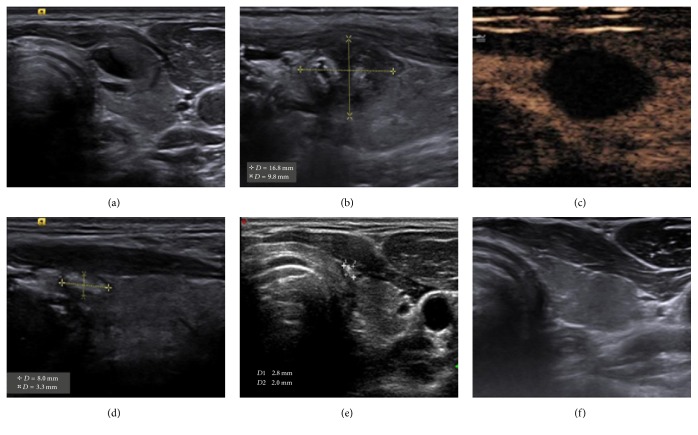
A 33-year-old Male had a mixed nodule in the left lobe of the thyroid. (a) Ultrasound examination showed a 13 × 8 mm mixed nodule protruding from the front capsule before LA. (b) Ultrasound examination showed a 16.8 × 9.8 mm ablation lesion one day after LA. (c) CEUS showed no enhancement of the ablation lesion one day after LA. (d) Six months after LA, Ablation lesion had an obvious volume reduction, and no prominent capsule was observed. (e) Nine months after LA, ablation lesion had an obvious volume reduction compared with the six-month results. (f) 12- month after LA, the ablation lesion had disappeared.
